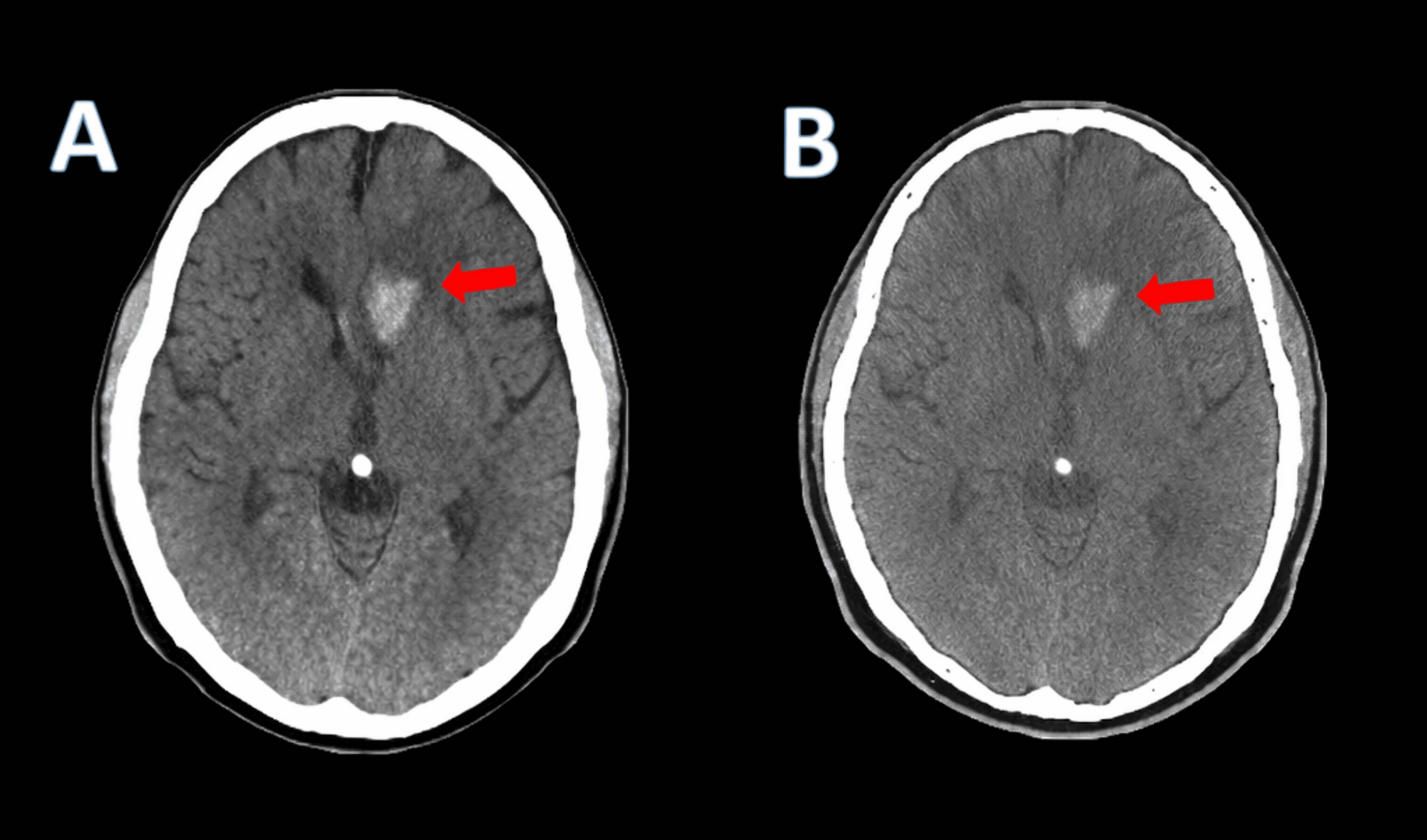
Non-contrast axial CT of the head. (A) Acute left frontal intraparenchymal hemorrhage with surrounding vasogenic edema (red arrow). (B) Adjacent intraventricular extension into the left frontal horn (red arrow).
